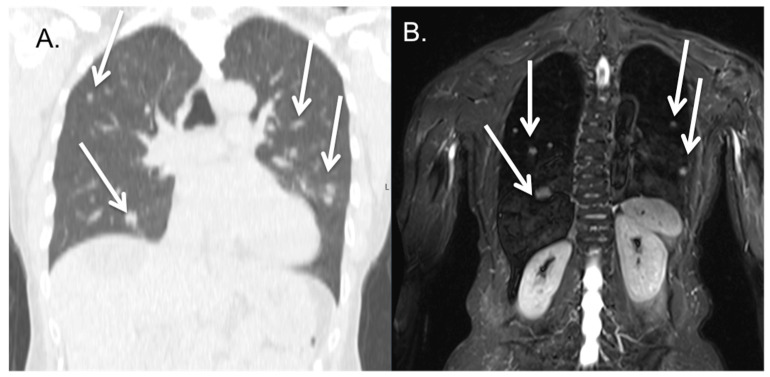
Patient with diffuse lung metastases; diffuse lung metastasis both visible on CT (A) and PET-MRI (B) in a patient with cervical cancer. Some of the matching lung metastasis are marked by a white arrow on both CT and MRI show matched results for both scans.
